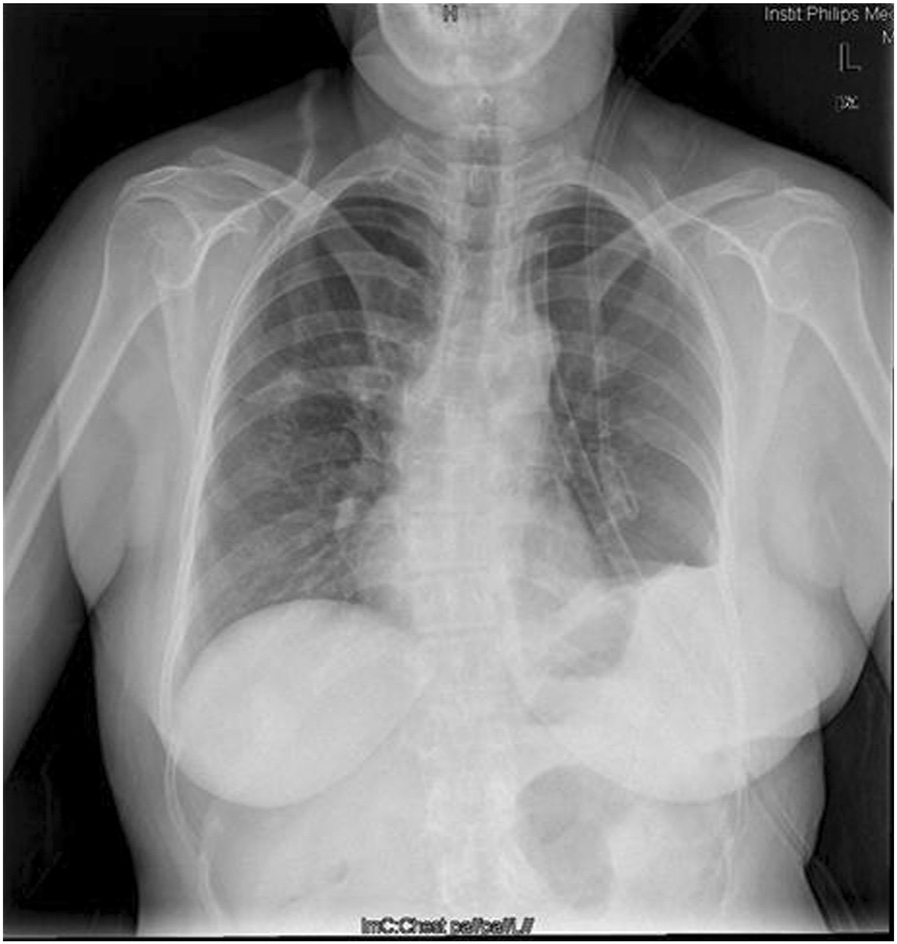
The repeated chest radiograph after 4 days of surgery showed the well recruitment of the left lung.
